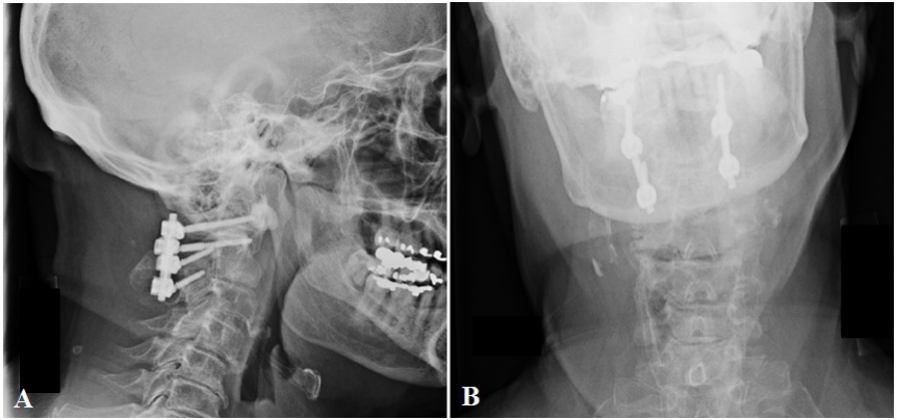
Postoperative X-ray Scans Postoperative X-rays (A, B) demonstrating removal of the sublaminar wires and proper hardware placement with fusion of cervical levels C1 and C2. Fusion was achieved using C1 lateral mass screws, C2 pars interarticularis screws, and rods.
